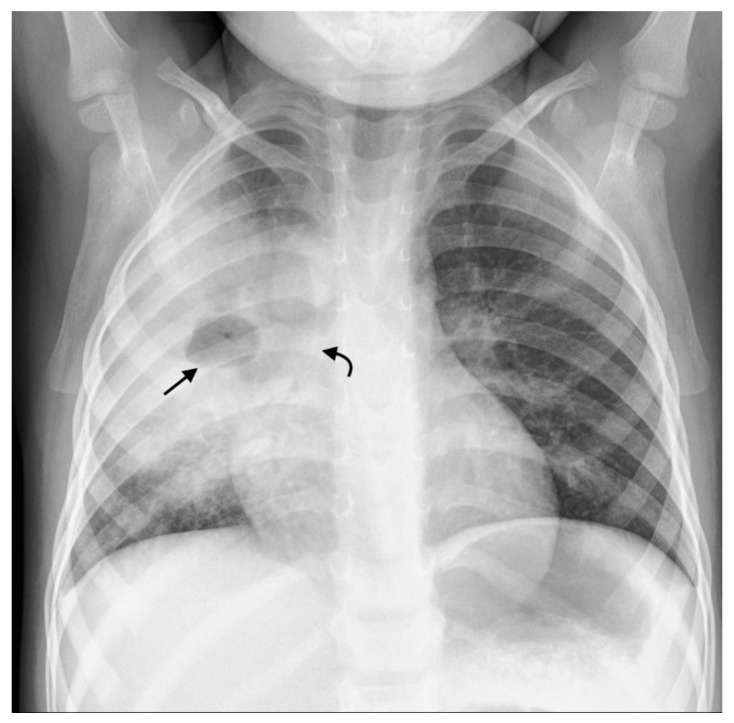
Frontal PA chest radiograph in a 3-year-old boy with confirmed pulmonary TB demonstrating right middle zone consolidation and cavitation (straight black arrow) containing an air-fluid level, as well as narrowing of the bronchus intermedius (curved black arrow).
